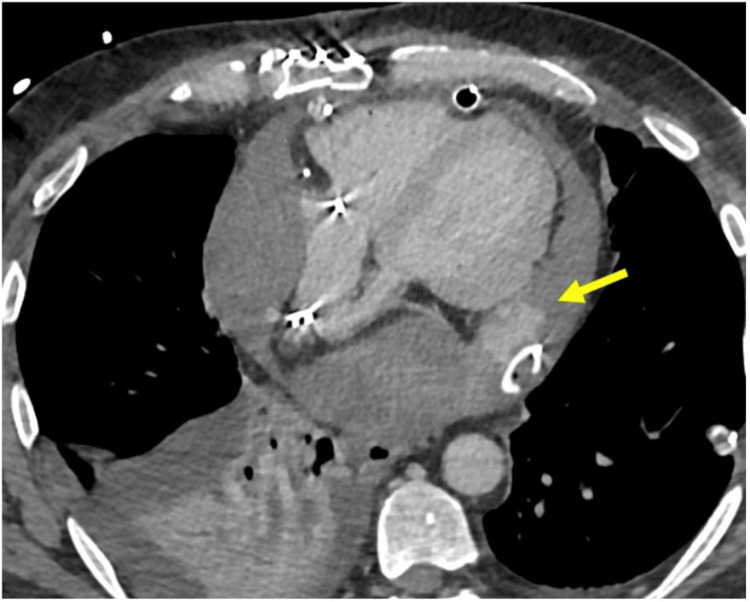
Delayed venous phase spiral CT angiogram showing active extravasation of contrast (yellow arrow) from a vessel on the posterior left ventricle, adjacent to the pericardial drain. This is more apparent compared to the arterial phase image at the corresponding axial slice.
